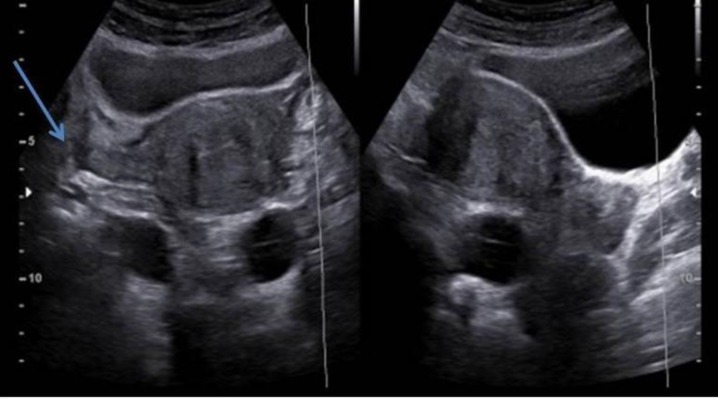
Axial and sagittal ultrasound plane shows normal uterus. Bilateral cystic structure posterior to the uterus is seen and ovaries are demonstrated separately. (Right ovary is detected in the axial image by arrow
